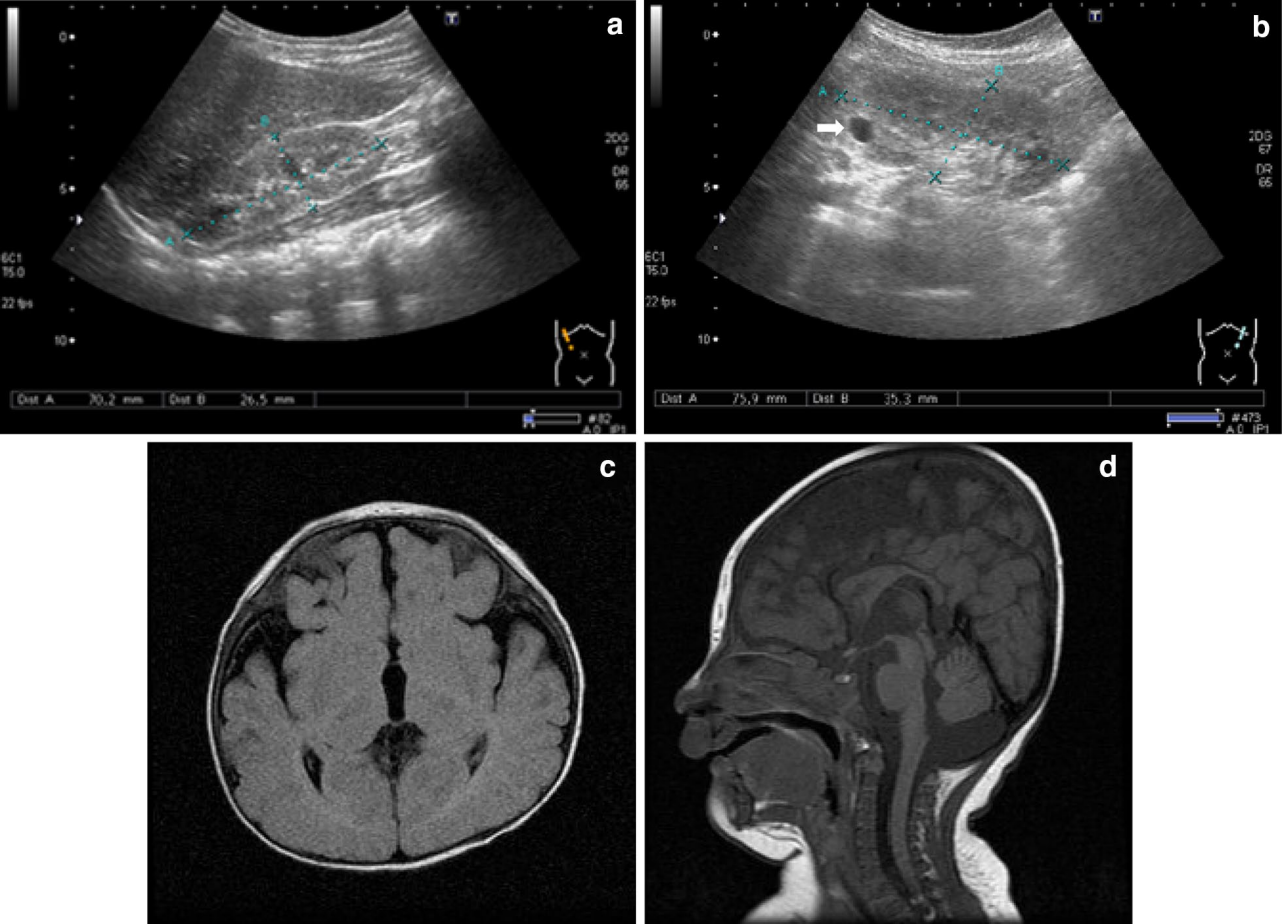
Ultrasonographic and magnetic resonance imaging examinations at 9 years and 4 months of age. Renal ultrasound showed the small size of the kidneys for the patient’s age: a the right kidney (length, 70.2 mm) and b the left kidney (length, 75.9 mm) with a simple renal cyst (1.13 × 0.78 cm) indicated by an *arrowhead*. Magnetic resonance imaging revealed brain atrophy; c diffuse enlargement of the subarachnoid spaces, bilateral widening of the sylvian fissures, and hypomyelination of the anterior white matter; and d thinning of the corpus callosum.
